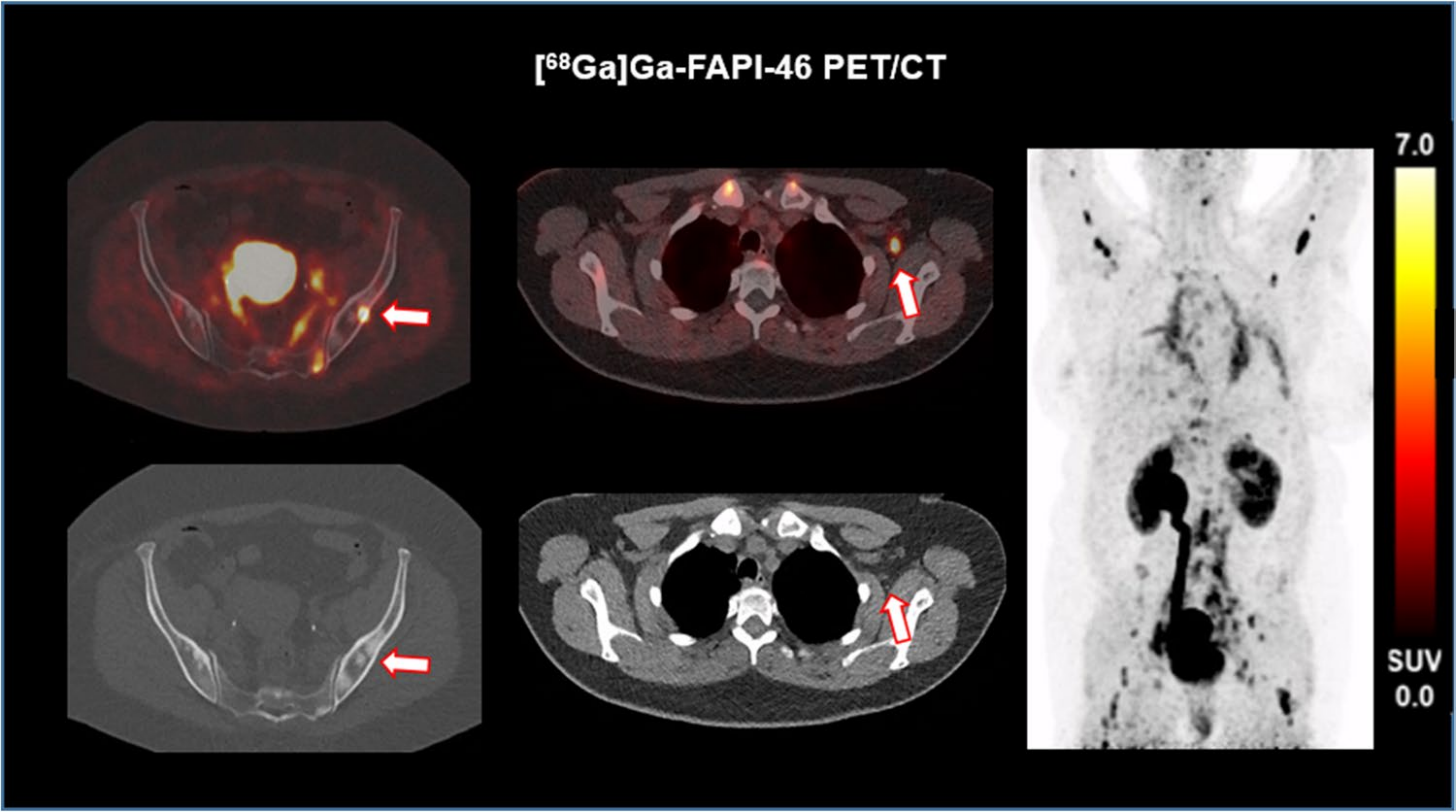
Increased [^68^Ga]Ga-FAPI- 46 expression in a patients with multiple bone and lymph node metastases who underwent radical cystectomy due to non-stoppable hematuria, Left: bone metastasis in the left ileum bone (SUVmax. 8.5, SUV_mean_ 7.9) (arrow); Right: left axillary lymph node metastasis (SUVmax. 9.9, SUV_mean_ 7.4) (arrow). The patient revealed an extensive nodal spread to mediastinum, left axilla, paraaortic and pelvic, pulmonary metastases and disseminated metastatic osseous spread
